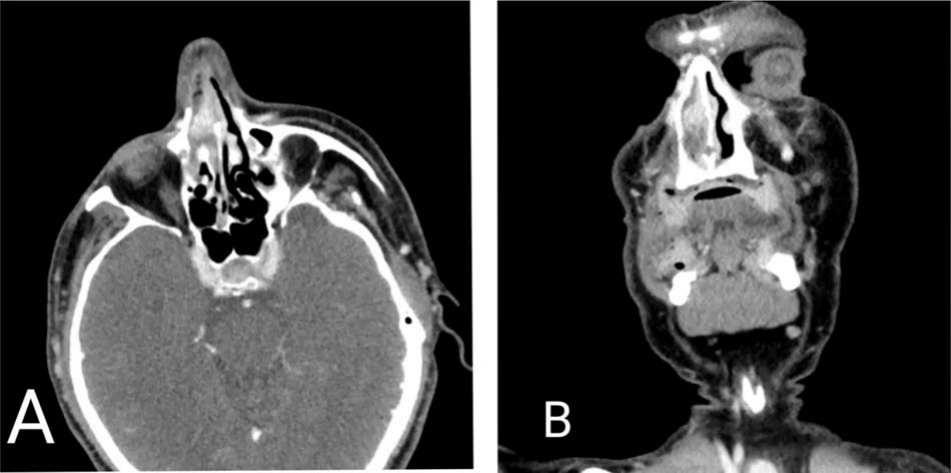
Computed tomography scan of the head. (A) Axial view and (B) coronal view demonstrating mass lesion in the right nasal cavity attached to the septum measuring 1 × 1.5 cm.
